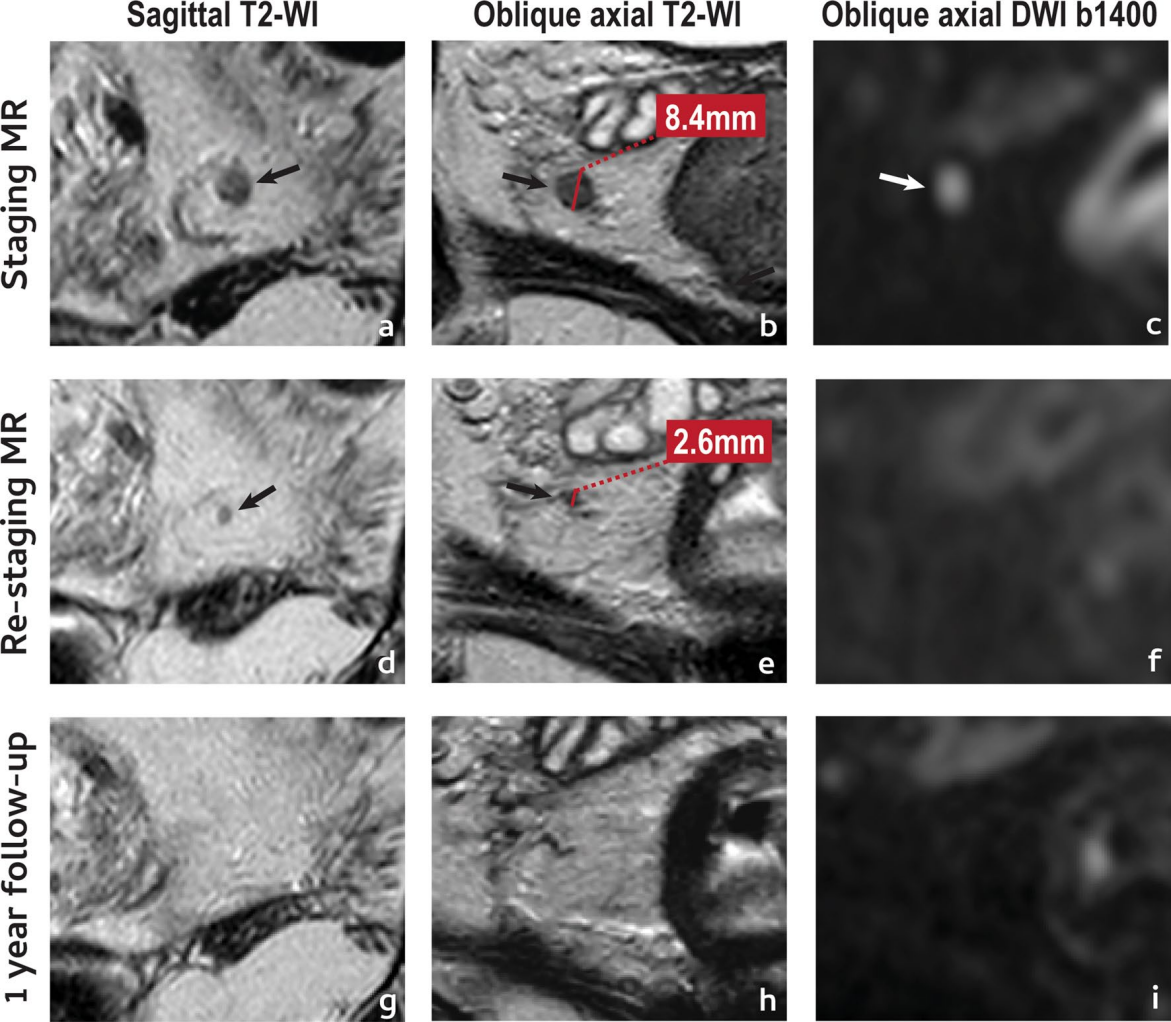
Complete lymph node response. A 42 year-old male presented with a low mrT3a (not shown) mrN1a (arrow in a–c) EMVI− CRM+ rectal cancer. He underwent NAT and was re-staged. The positive lymph node showed a pronounced reduction in size after NAT, with a short axis reduction of 70% and a short axis < 5 mm (e). On T2-WI, the lymph node became too small to characterise (e). The lymph node was not visible on re-staging high b value DWI (f) and disappeared also on T2-WI at 1-year follow up MR imaging (g–i). Patient is currently under W&W with no clinical signs of viable local or distant disease
